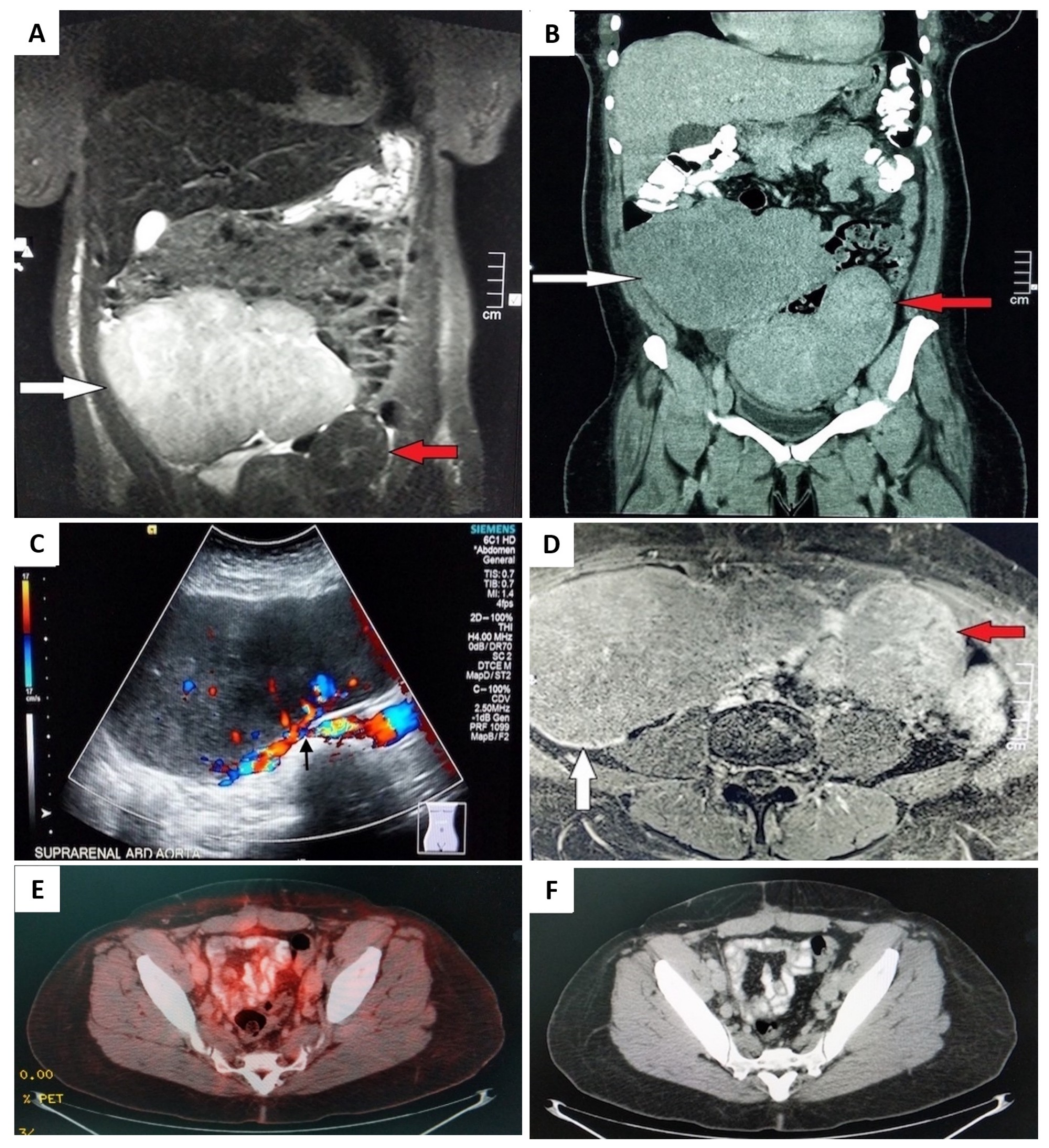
Radiology images at the time of presentation. A coronal T2W MRI (A) and coronal CT (B) images demonstrating an exophytic mass (red arrow) arising from the uterus (leiomyoma) and a large tumor mass (white arrow) with high signal intensity on MRI. A trans-abdominal ultrasound (C) demonstrating a large tumor mass with increased vascularity (arrow). An axial T2W MRI image (D) showing a large tumor mass (white arrow) and left tumor mass (red arrow). Post-therapeutic radiology images (E, F): A PET/CT image (E) showing no evidence of hypermetabolic activity. An axial CT of the pelvis (F) showing the post-surgical empty space in the center of the pelvis occupied by the bowel with no evidence of malignancy. Abbreviations: T2W, T2-weighted; PET, positron emission tomography.
